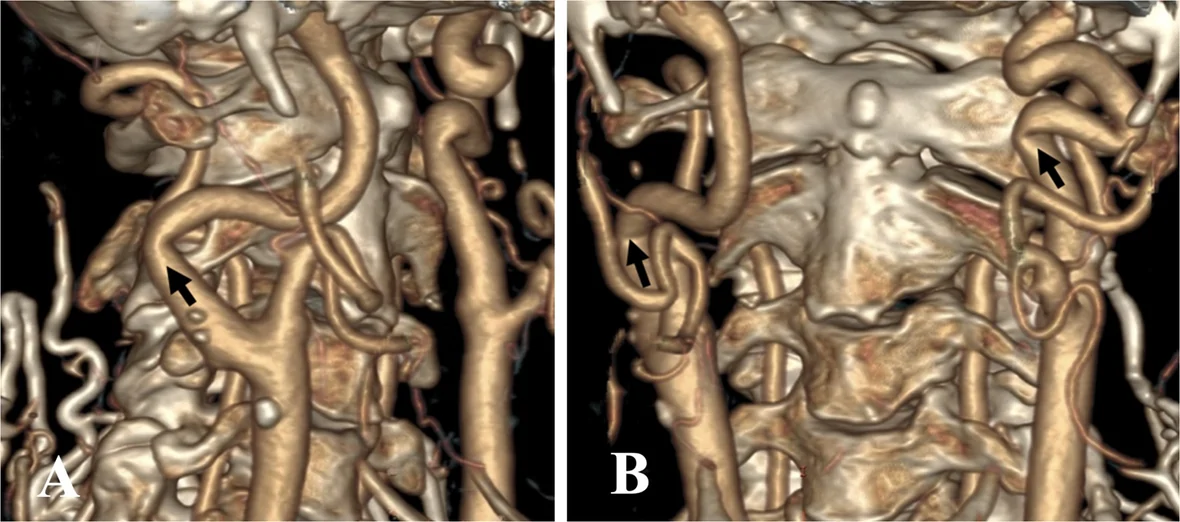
CT angiography showing the tortuous pattern (black arrow) of the cervical portion of the internal carotid artery. A. Sagittal view, B. Frontal view
